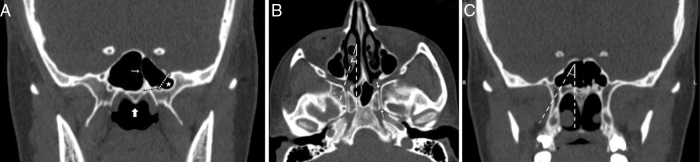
Morphometric measurements (A) Coronal CT sections showing the distance from the vidian canal to palatovaginal canal (black dotted line) and the foramen rotundum (white dotted line), the septum (thin arrow) between the vidian canal and vomerine crest (thick arrow), the pterygoid process pneumatization (star). (B) Axial CT section showing the angle between the posterior end of the middle turbinate and lateral margin of the anterior opening of the vidian canal, the anterior opening of the vidian canal (thick arrow) and the posterior opening of the vidian canal (thin arrow). (C) Coronal CT sections showing the angle between the posterior end of the middle turbinate and lateral margin of the anterior opening of the vidian canal.
